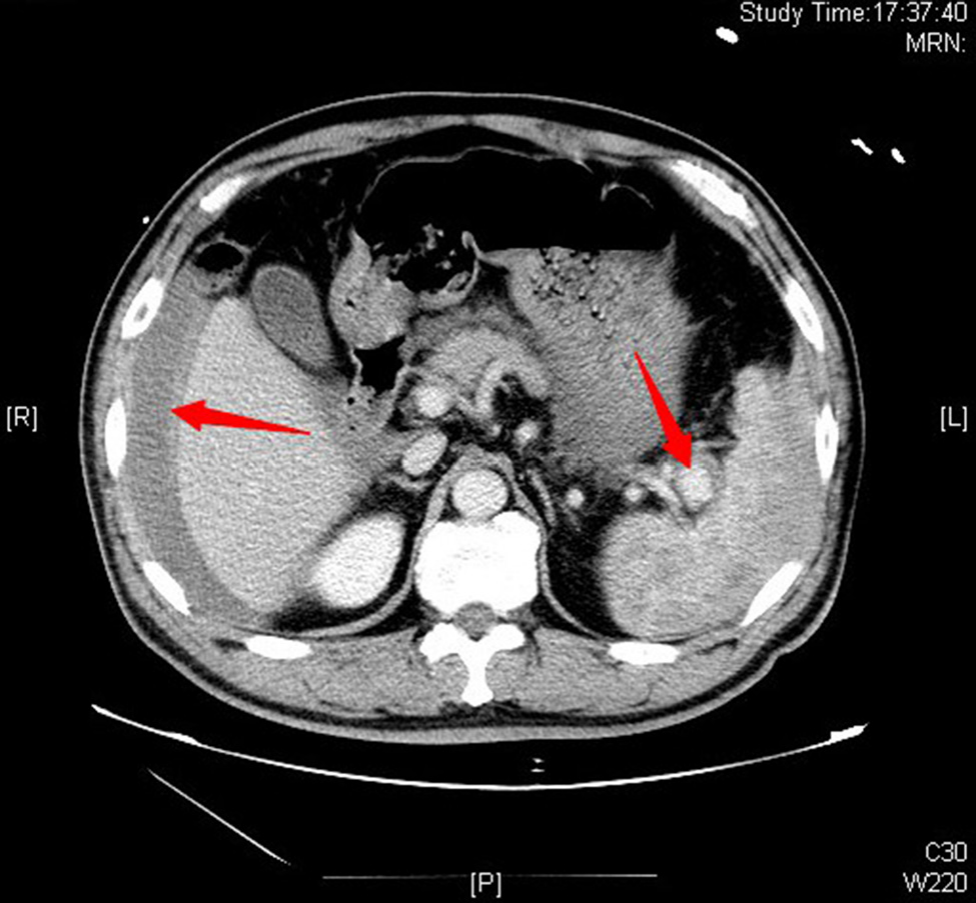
Contrast-enhanced CT of the abdomen showed perihepatic hemorrhage and splenic aneurysm (arrows).
